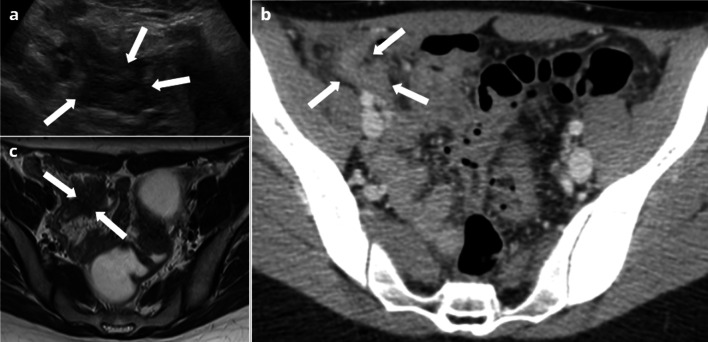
26-year-old female presenting with acute right lower quadrant pain. US and CT (a, b) show an irregular pseudonodular hypoechoic (a) enhanced (b) thickening of the appendix (arrows), with pelvic fat stranding. T2W MRI (c) shows a fibrotic endometriotic implant of the right ovarian fossa, invading the appendix (arrows). Surgery was performed and found hemoperitoneum along with many endometriotic implants of the ileocecal region. Pathological reports found endometrial stroma in the muscular layer of the appendix
